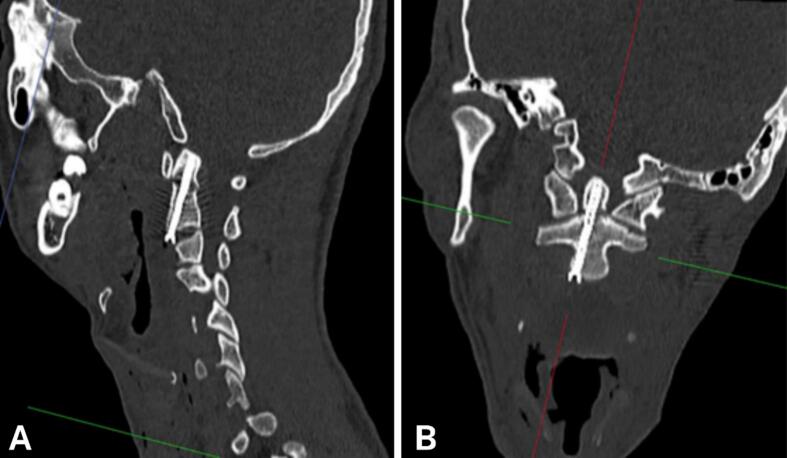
CT scan 6 months after surgery shows that the screw is still in good position, not loose or displaced.
